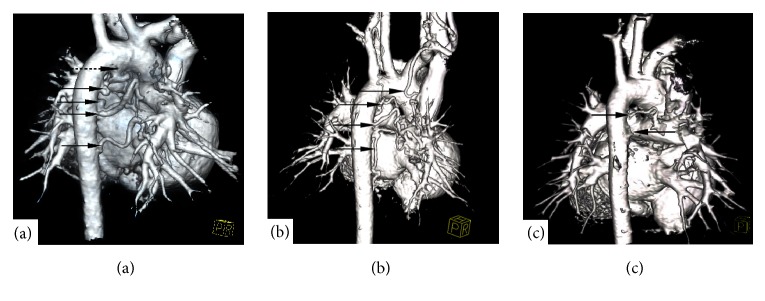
CTA images of 3 typical patients with MAPCAs of different K values (collaterals marked by arrows): (a) the patient with K≥2 (2.28), and one large collateral was ligated during operation (dotted arrow). (b) The patient with 1<K<2 (1.60). (c) The patient with K≤1 (0.62).
